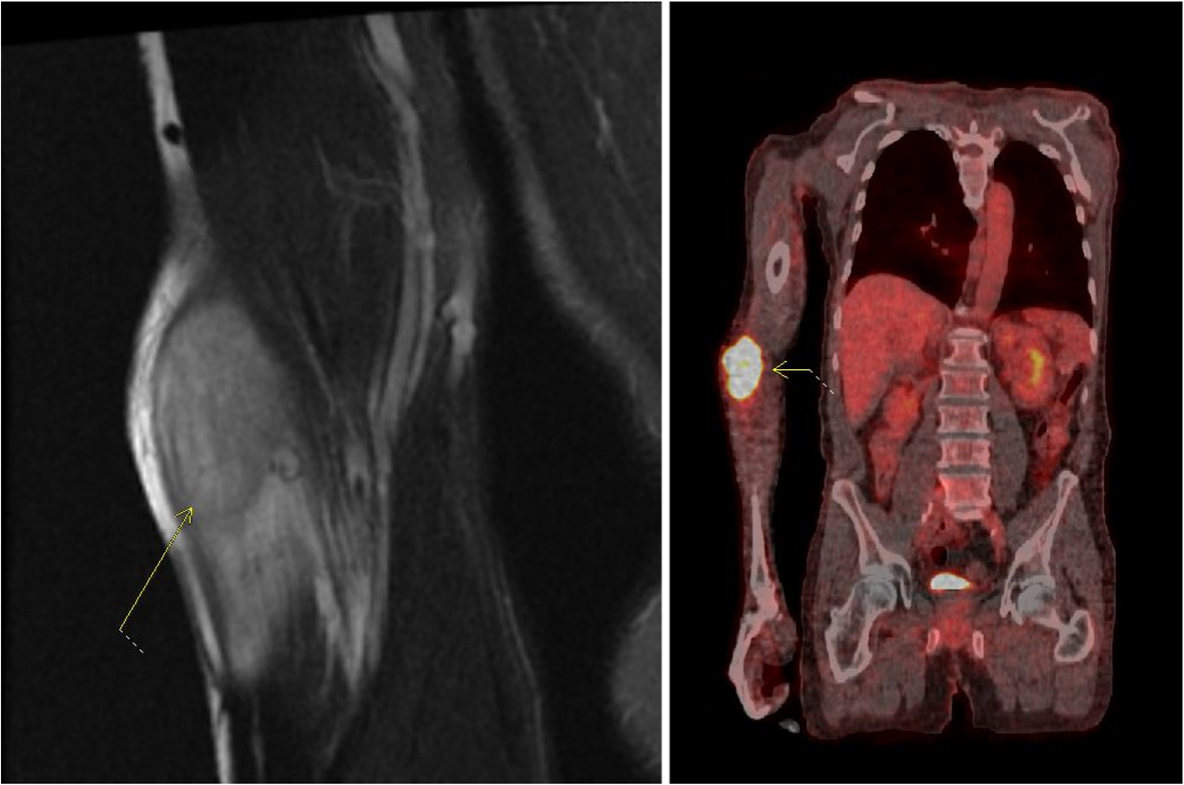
MRI and PET scan imaging of right elbow mass, 4 months post-operatively
